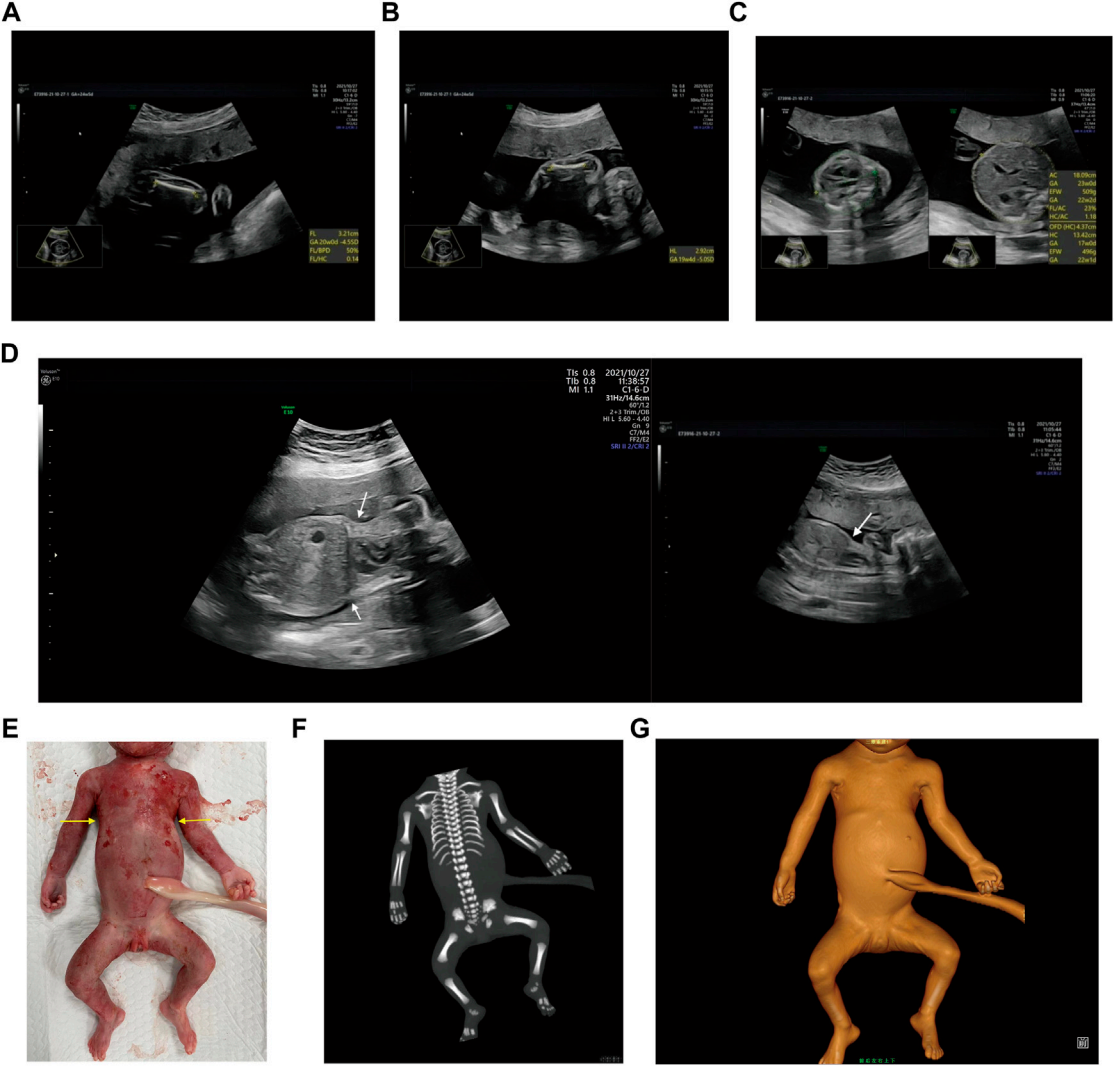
Antenatal ultrasound, photos and radiographs of the foetus 1 (A) The femur length was 3.2 cm. (B) The humerus length was 2.9 cm. (C)The thoracic circumference and abdominal circumference was 13.4 and 18.1 cm respectively. (D) Sagittal view of fetal thorax and abdomen showed a “thoracoabdominal notch” in the junction of thorax and abdomen (indicated by the arrows). (E) Appearance of the foetus after induced labor. A narrow thorax was observed. (F, G) Radiographs and CT 3-dimensional image reconstruction of the foetus. A narrow thorax and short long bones were observed.
